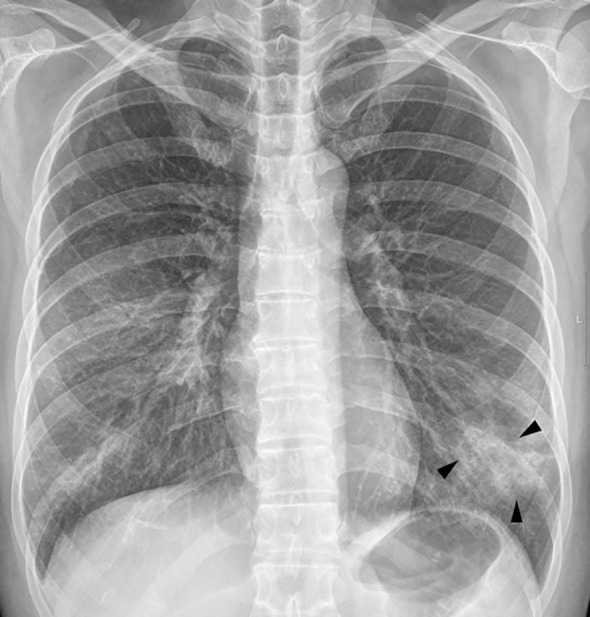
Chest radiograph at initial admission. Posteroanterior chest radiograph obtained on hospital day 1 shows consolidation in the left lower lung zone. A subtle ground-glass opacity (GGO) is also visible in the right middle–lower lung zone.
